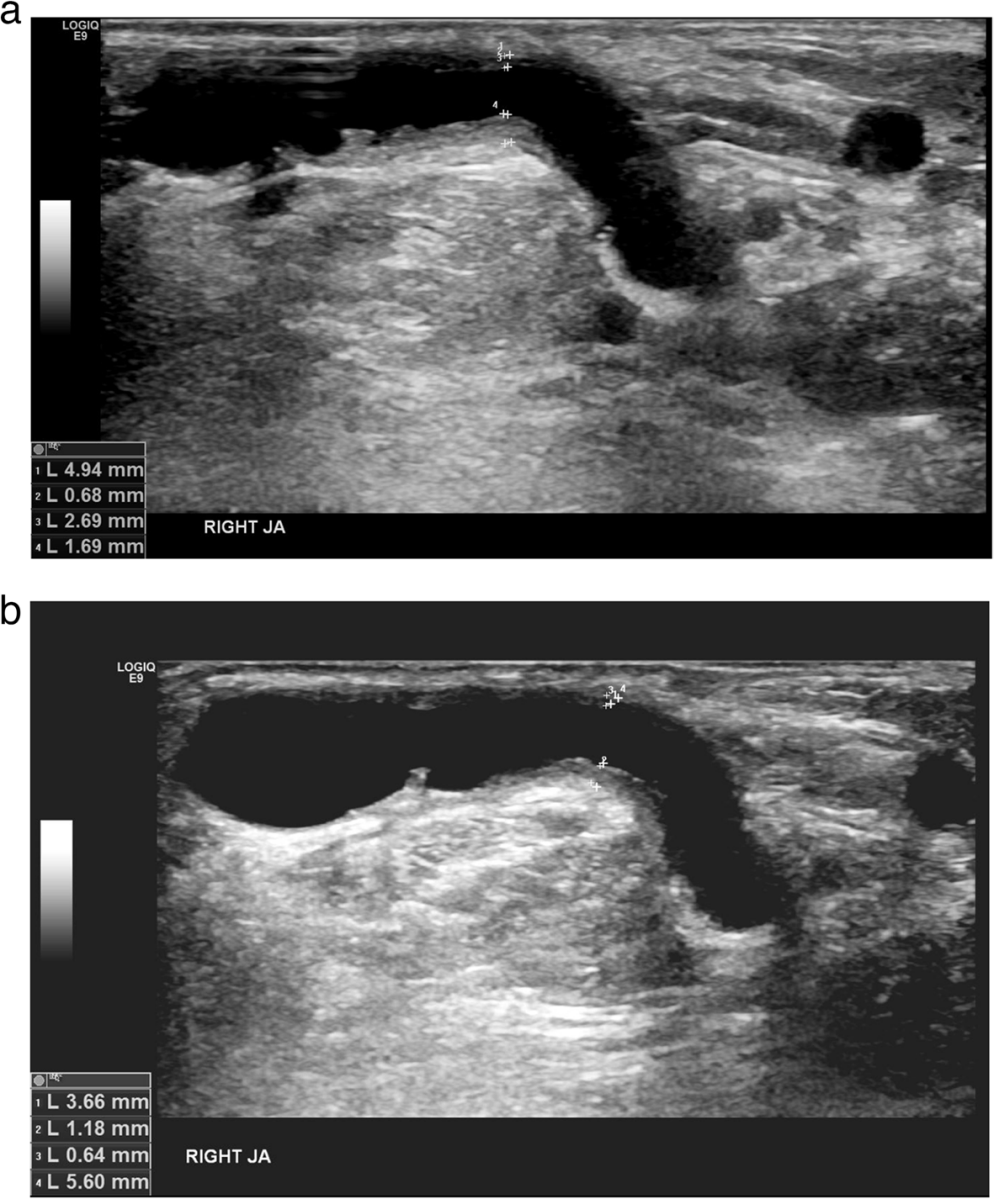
a, b B mode ultrasound images of a juxta-anastomotic stenosis pre (a) and 59 days post DCB angioplasty (b). %IMT has reduced from 50 to 33%
